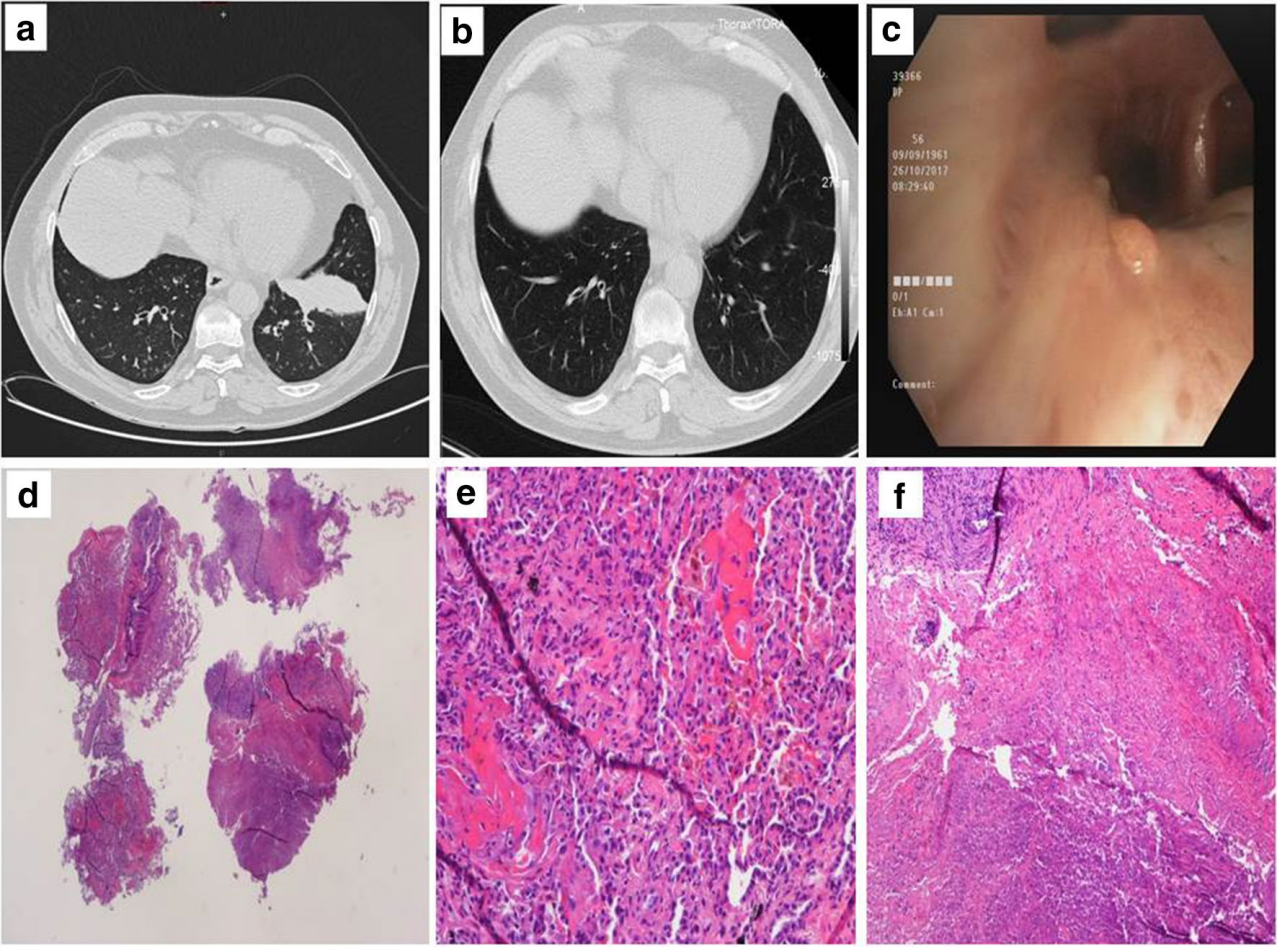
a CT image showing the presence of varicoid bronchiectasis and parenchymal infiltration around the basal anterior segmental branch line of the left lower lobe. b CT image showing resolution of parenchymal opacity. c Fibreoptic bronchoscopy showing two small nodular neoformations in the sub-segmental branches of the lower right lobe. d Biopsies (×20 magnification): on the right there are two fragments with necrosis, on the left two fragments with haemorrhage, in the form of blood with fibrin, hemosiderin and organizing pneumonia. e and f Necrosis and bleeding are best seen at higher magnification, ×200 left and ×100 right, respectively
